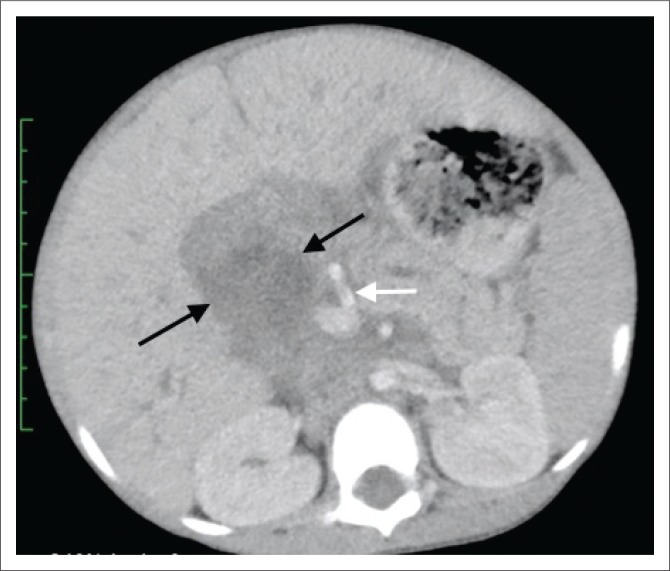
Axial post contrast computed tomography image at the level of the origin of the superior mesenteric artery (white arrow) demonstrating a fusiform-shaped mass (black arrows) with heterogeneous contrast uptake, parallel to the expected course of the common bile duct. Associated findings of prominent intrahepatic bile ducts and a dilated pancreatic duct.
